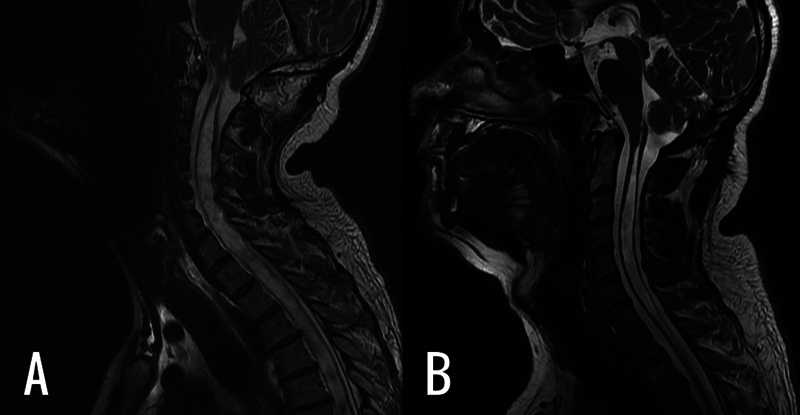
Brain and cervical magnetic resonance imaging (MRI) scan showing herniation of the cerebellar tonsils through the foramen magnum and dilation of the central canal of the spine associated with syringomyelia, both findings of Chiari type I malformation, which is classically related to spontaneous downbeat nystagmus (DBN). (
